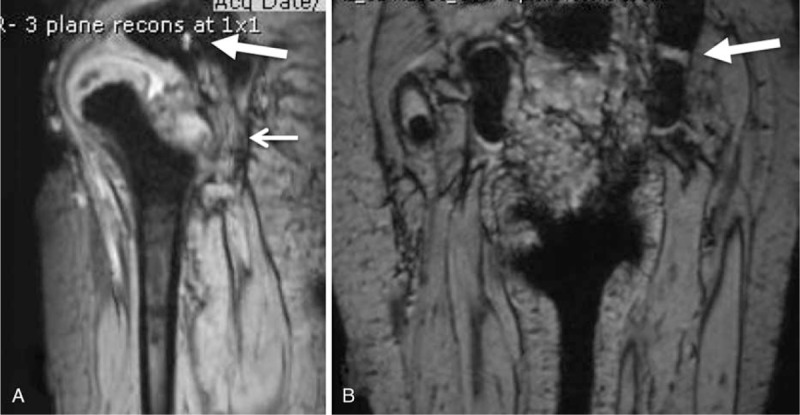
T2-weighted MRI (A) Sagittal MRI with thick arrow pointing to the anomalous foramen and the thin arrow at the course of the sciatic nerve. (B) Coronal MRI with thick arrow at the anomalous foramen containing the sciatic nerve. MRI = magnetic resonance imaging.
